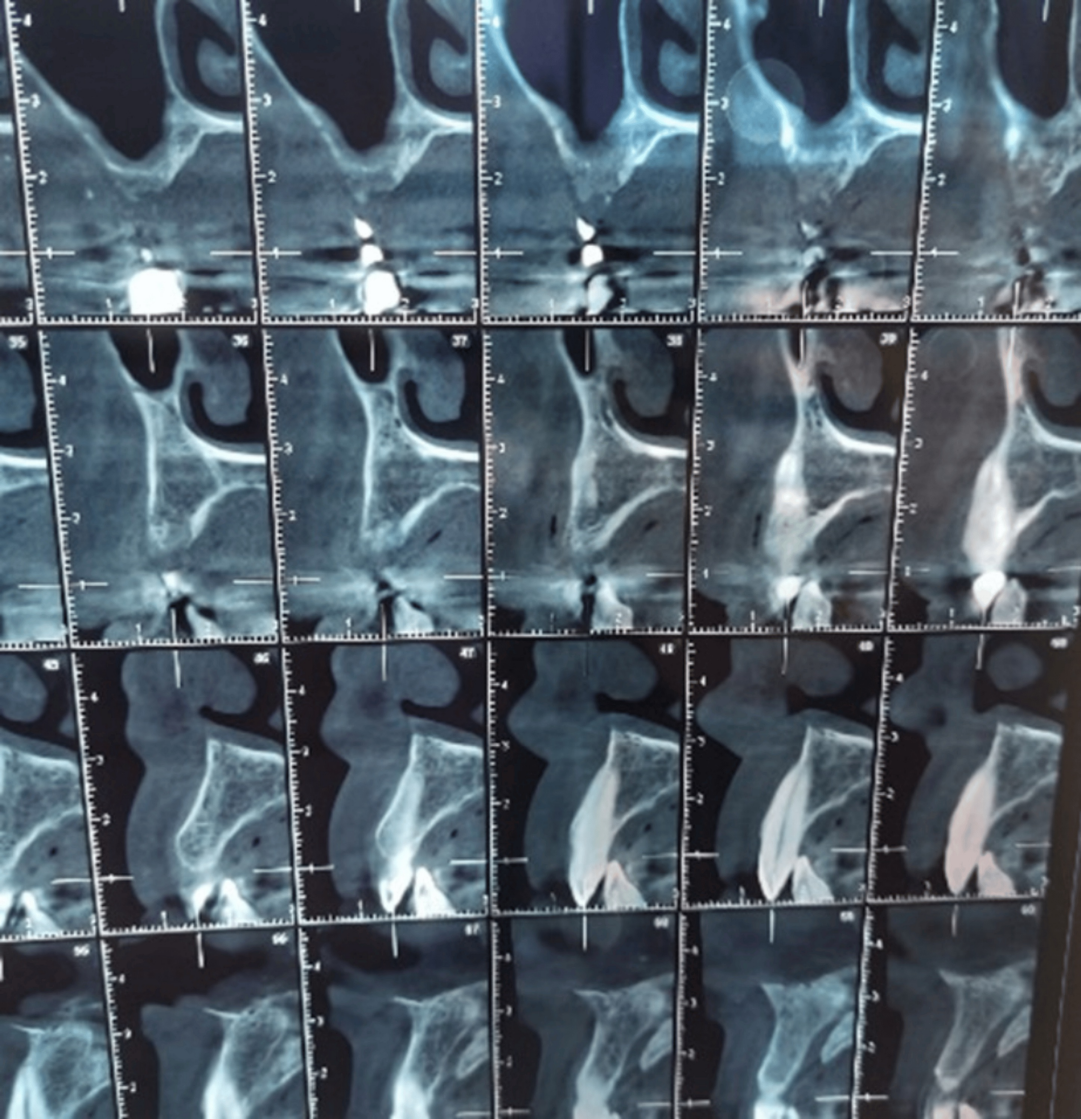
CBCT evaluation. An oblique coronal CBCT view showing a low bone height except at the site of the 14.
